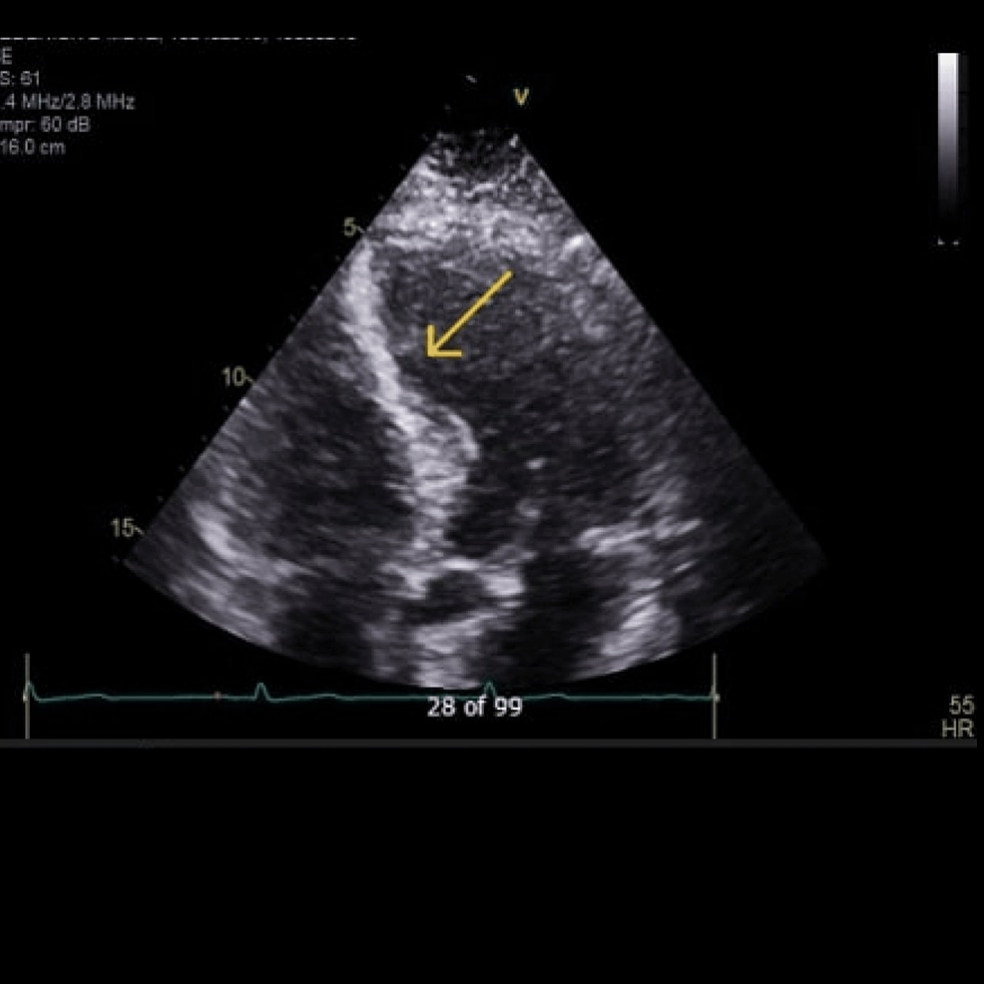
Echocardiogram from May 2021. Yellow arrow pointing to the interventricular septum. Transthoracic echocardiogram (TTE) complete with contrast, bubble, strain, and 3D PRN order panel (May 2021). Acquisition window and orientation are an apical four-chamber view, utilizing Change Healthcare Cardiology Software (CPACS) as the imaging tool/imaging mode. Left ventricle (LV) cavity size is normal with mild concentric hypertrophy. There is grade I (mild) diastolic dysfunction and normal left atrial pressure. Visually estimated ejection fraction is 65%. There are no LV wall motion abnormalities noted. LV mass and mass index (2D) are 179 g and 77 g/m^2^, respectively. Left ventricle wall thickness is noted as normal. LV outflow tract (LVOT) stroke volume and LVOT stroke index are 139 g and 60 mL/m^2^, respectively. No significant changes were observed when compared to previous TTE imaging. Imaging obtained from centralized electronic medical records with the consent of the patient.
